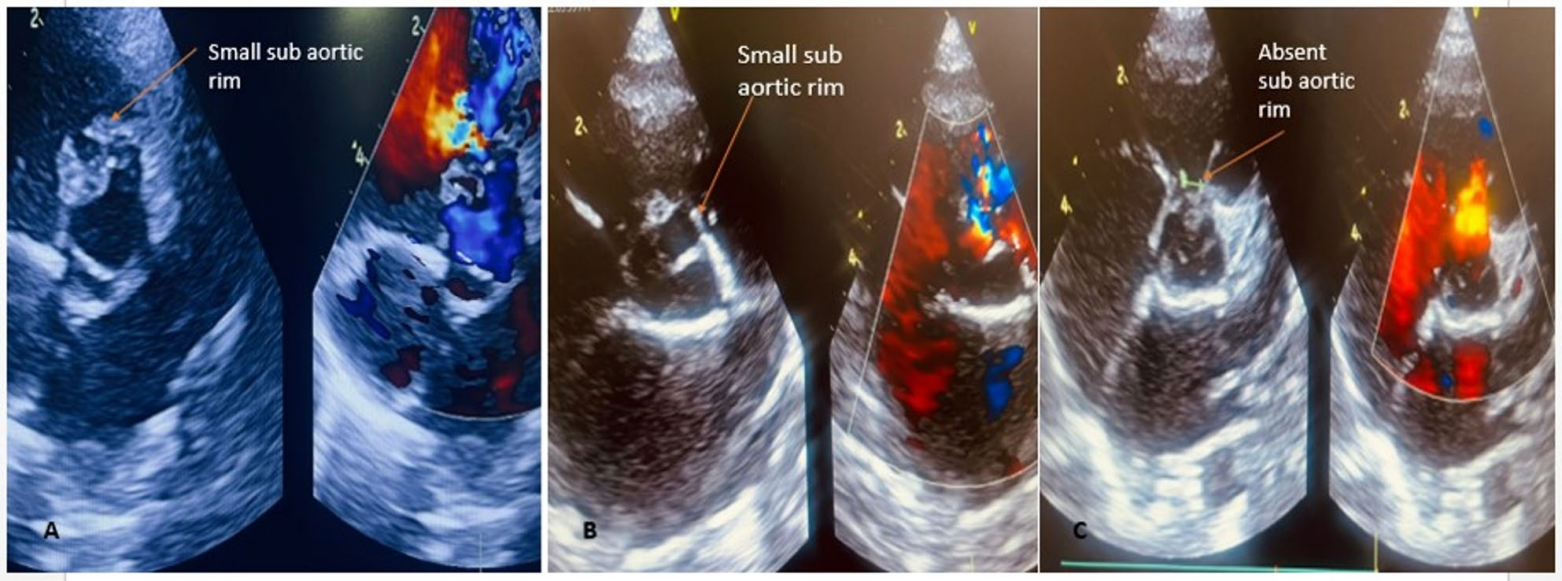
Echocardiographic profiling of outlet VSD in parasternal short-axis view, with A, B showing (arrow) presence of small subaortic rim in OVSD, C showing (arrow) an absent subaortic rim (not suitable for transcatheter closure)
